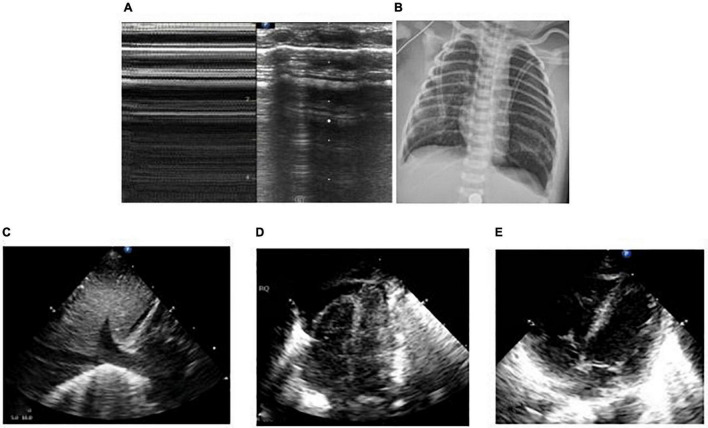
The patient was born at 39 weeks of gestational age by natural delivery. The amniotic fluid was III turbid, and the Apgar score of 1, 5, and 10 min of birth were 2, 3, and 6 points. Respiratory distress occurred after birth and requiring mechanical ventilation. It was diagnosed as neonatal meconium aspiration syndrome. Under mechanical ventilation, man-machine incongruity appeared. The patient had weakened breath sounds in both lungs, a heart rate of 120 beats/min, dull heart sound, pale skin tone, cool limb ends, and weak radial artery beats. The blood pressure was 56/35 mmHg, and the shock score was 5 points. Continuous hypoxemia appeared, the arterial blood gas was PO_2_ 30.4 mmHg, and calculated Oxygenation index (OI) value was 50. Emergency bedside ultrasound revealed the lung slip of both lungs disappeared and without B-line, stratospheric sign showed, and the lung point was not explored, suggesting the diagnosis of massive pneumothorax on both sides (A). Emergency thoracocentesis was performed under ultrasonic monitoring. After the operation, the blood oxygen saturation increased, blood pressure rose to 65/40 mmHg and the heart sound was strong. After 30 min, the X-ray operator at the bedside arrived, and the X-ray chest film showed bilateral pneumothorax (B). In emergency situations, bedside ultrasound is easier to obtain than X-ray examination. In the figure, who has massive pneumothorax on both sides, hours after thoracic puncture and drainage, the baby appeared to cyanosis, the breath sounds in both lungs was weak, the heart rate increased to 180 beats/min. Reexamination of LUS showed that pneumothorax decreased and the lower boundary of lung was too low, bedside cardiac ultrasound showed inferior vena cava expansion and fixation (C), ventricular filling and relaxation limitation (D), suggesting that the patient had excessive lung expansion. The ventilator pressure was reduced to increase the returning blood volume, and the heart rate decreased gradually to 150 beats/min. Ventricular filling and diastolic improvement were observed by ultrasound (E).
